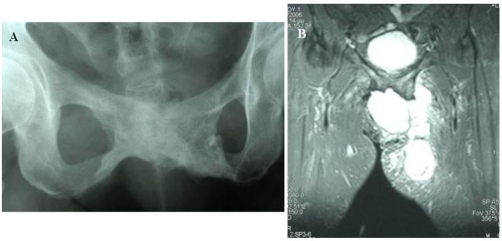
Preoperative plain X-ray (A) and MRI imaging of the tumor (B).
